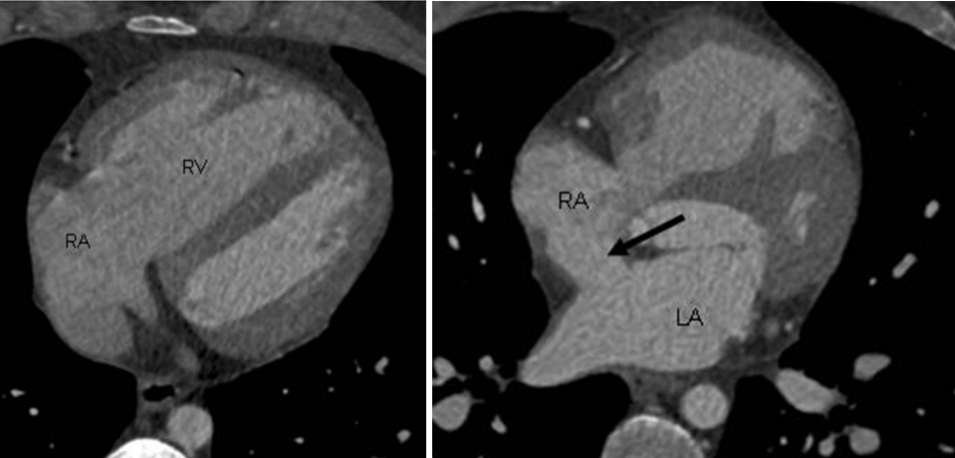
A dilated right ventricle of the heart and superior sinus venosus atrial septal defect (ASD). A male patient with pulmonary hypertension and right-sided volume overload. Multidetector computed tomography documented a dilated right side of the heart (a) and a large superior sinus venosus ASD (b), which could not be confirmed with transthoracic echocardiography. RA = right atrium, RV = right ventricle, LA = left atrium.
